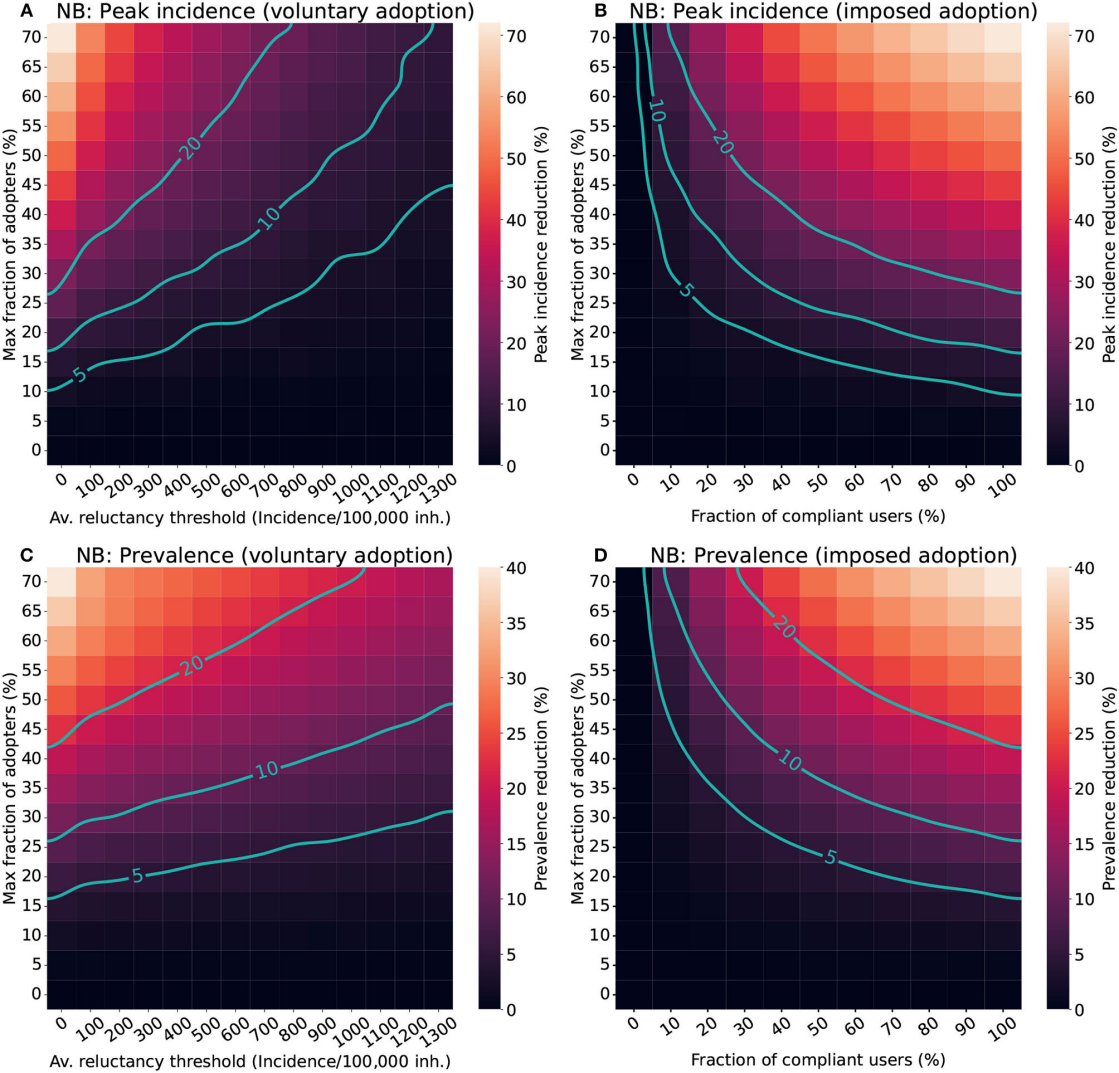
Impact of different factors of human behavior in the effectiveness of CT apps in a population with a Negative binomial distribution (NB). For the voluntary adoption scenario (A, C), the parameters explored are the average reluctance threshold and the maximal fraction of adopters. Meanwhile, in the imposed adoption scenario (B, D), changes in the fraction of cooperative users and the maximal fraction of adopters are explored instead. The color scale reflects the average reduction produced by the CT app (Δ) in the peak incidence (top panels) or maximal prevalence (lower panels). The isoclines indicate the combinations of parameters resulting in Δ = 5%, Δ = 10%, and Δ = 20%.
